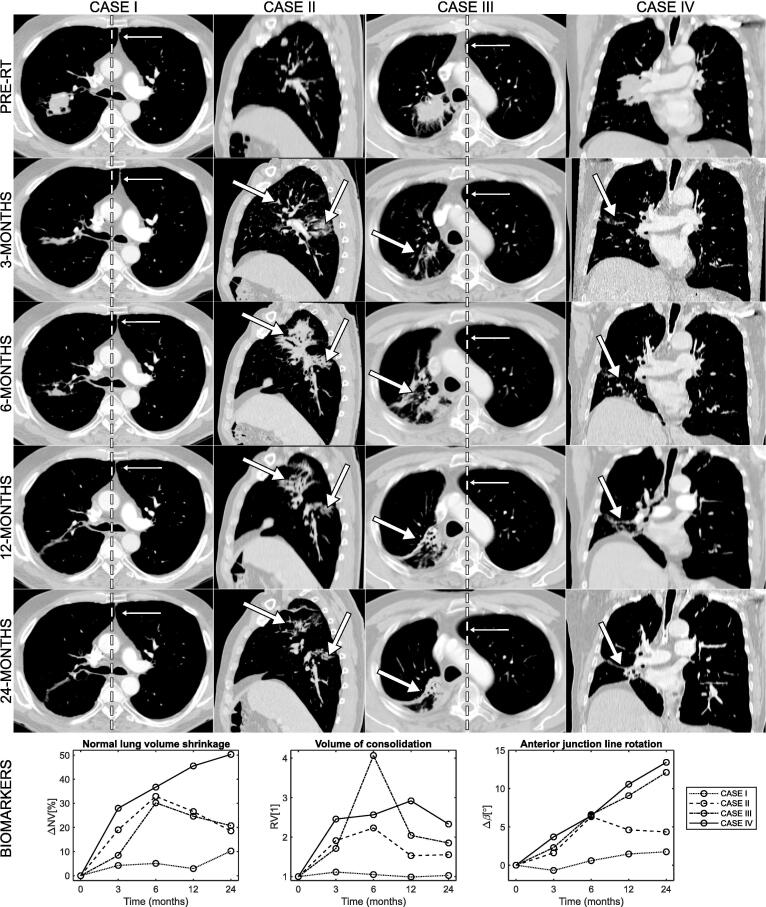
Examples of evolution (from pre-RT to 24-months post-RT) of radiological changes and corresponding values calculated for selected CT-based biomarkers. Case I: The observed changes, which included parenchymal damage, lung volume shrinkage and anatomical distortions, were mild but measurable, and worsened over time. The dashed line highlights the evolution of rotation of the anterior junction line (Δβ) indicative of volume loss with anatomical distortions. Case II: Diffuse parenchymal change was visible at 3-months (arrow); at 6-months those regions evolved into dense consolidation. Most parenchymal changes (RV) visually resolved by 24-months. Anatomical distortions were modest and did not worsen after 6-months (Δβ). The pronounced loss in normal lung volume (ΔNV) at 6-months was explained primarily by inflammatory changes and was not permanent. CASE III: Normal lung volume loss and consolidation volume peaked at 6-months (ΔNV and RV). As time progressed, the regions of consolidation shrank and became denser while anatomical distortions were increasingly evident (Δβ). The observations reflecting inflammation tended to resolve whilst those reflecting scarring progressed. CASE IV: Lung volume loss progressively worsened with time (ΔNV), with anatomical distortions also becoming more apparent as time passed (Δβ). This is compatible with a dominant effect of permanent fibrotic scarring. The increase in high-intensity lung volume remained stable across time-points although there was progressive lung volume shrinkage (RV and ΔNV). This was due to the presence of a residual mass that shrank across follow-up time-points in parallel with the scarring process. Complementary projections in supplementary material B (Fig. S.2).
